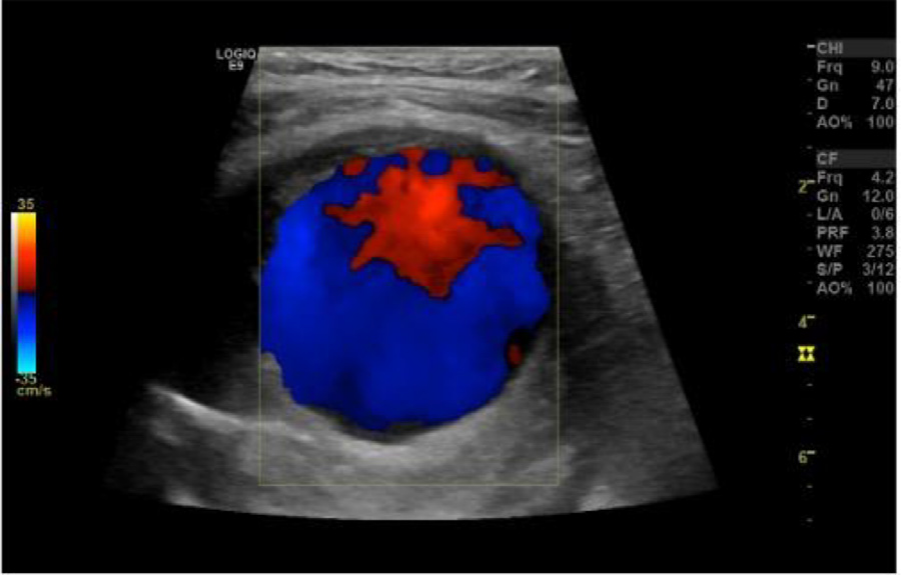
Duplex sonography of the popliteal artery. Legend 2: Duplex ultrasonography shows extraluminal blood flow of the popliteal artery.
